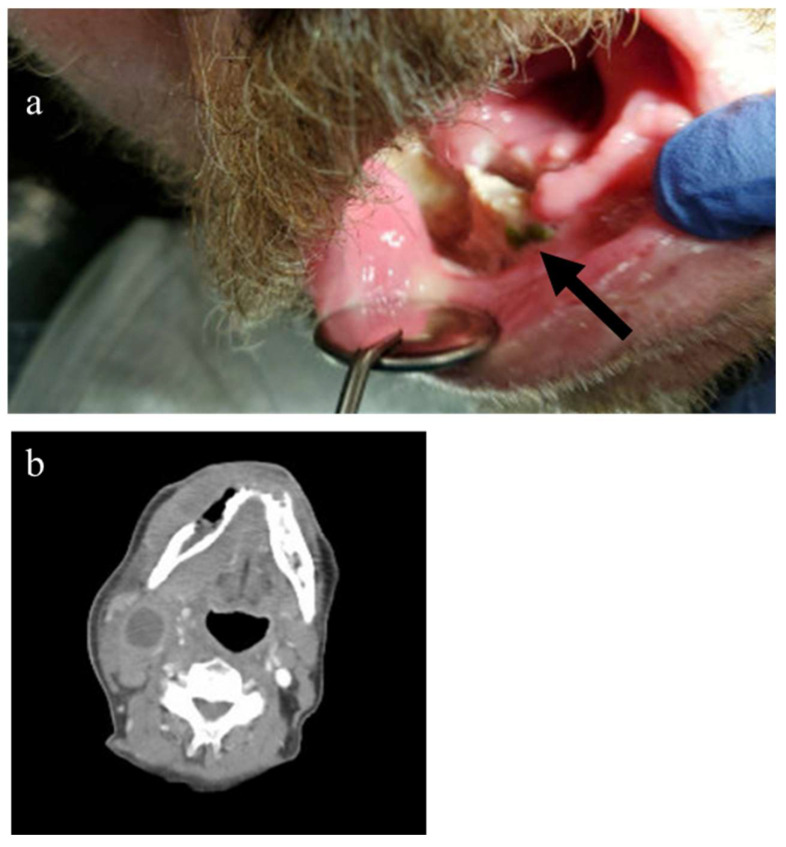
A 64-year-old man with DLBCL stage IIeb at the time the lymphoma diagnosis was made, 2 months after 1st biopsy and after no improvement with systemic antibiotics. (a) Photo of right lower oral cavity site of tumor (black arrow). (b) CT scan taken during staging, with mass and associated bone destruction in yellow circle and necrotic cervical nodes red arrow.
